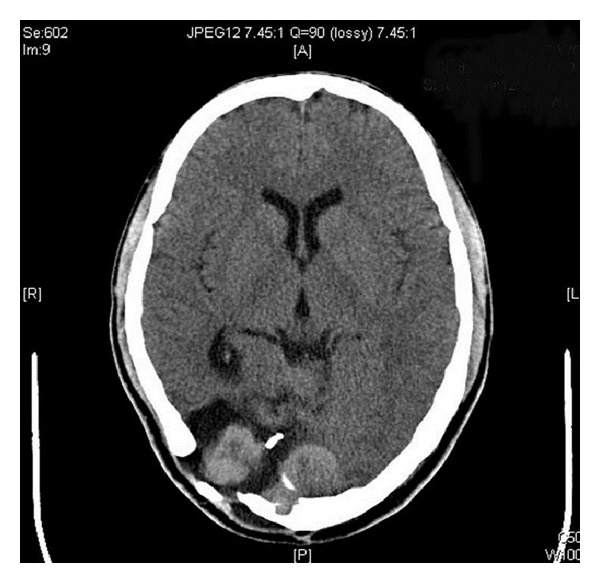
MRI scan demonstrates the recurred tumor mostly involved right posterior fossa.
